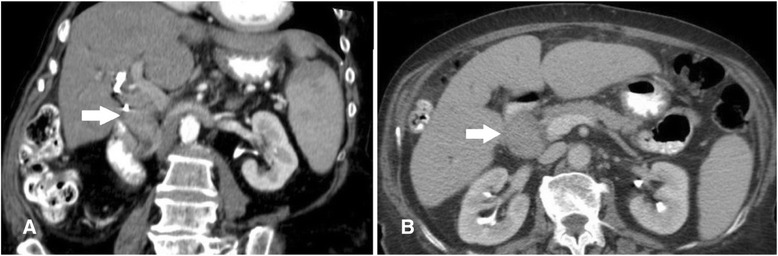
Computed tomographic scan shows the presence of common bile duct–obstructing homogeneous mass (*arrows*). a Coronal view. b Axial view
